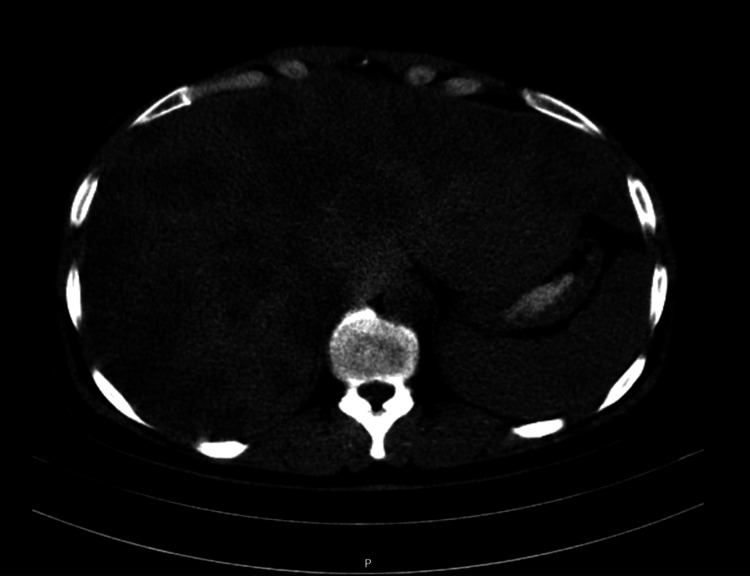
Axial CT image at diagnosis (2009) showing extensive multinodular hepatic metastases.
